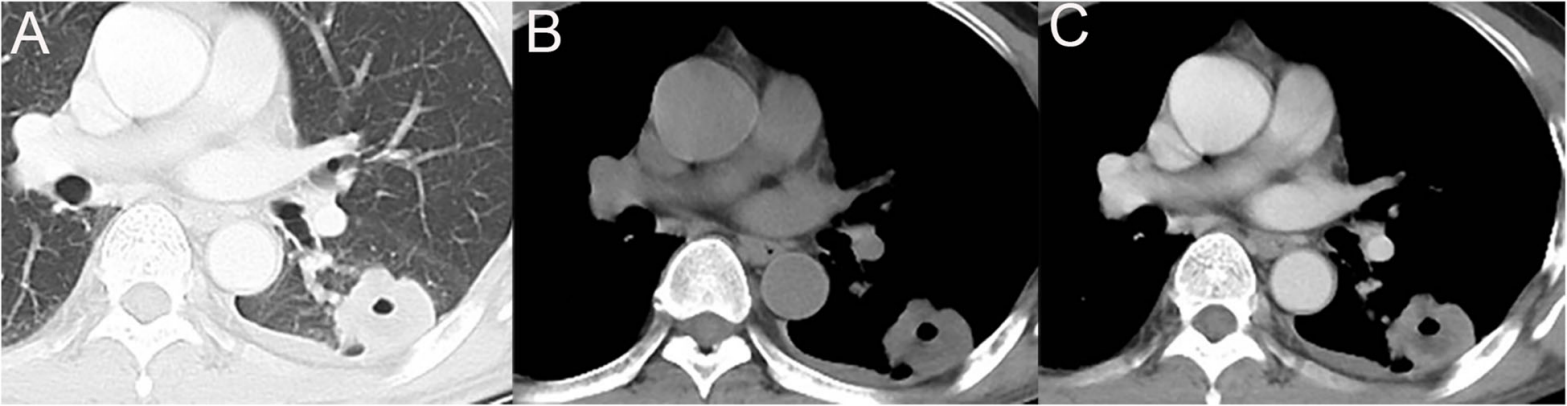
A PMEC with irregular shape and ill-defined margin with peripheral type. Axial non-contrast CT image (a,b) shows a hypodense mass (arrows) in left inferior lobar dorsal segment. Contrast-enhanced CT image (c) shows tumor with moderate enhancement and necrosis (arrows)
